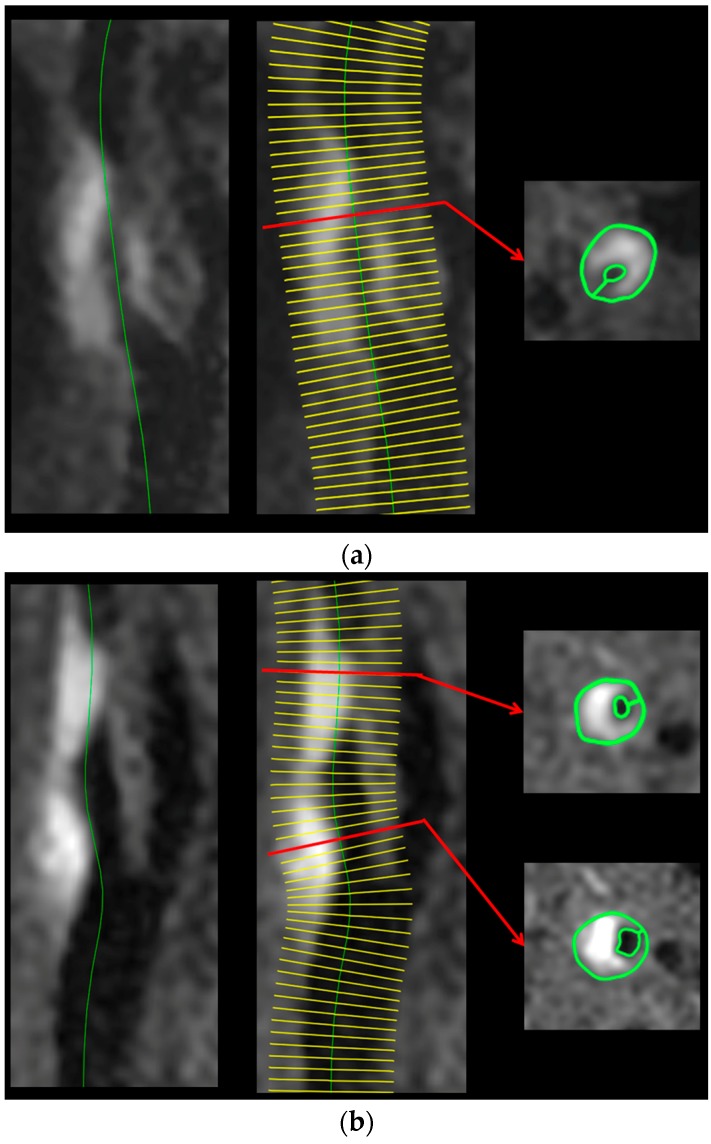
Preoperative three-dimensional fast spin echo T1-weighted magnetic resonance (3D FSE T1-weighted MR) plaque images of two patients with MES during exposure of the carotid arteries in endarterectomy. (a) The curved planar reformation is performed parallel to the long axis of the common and internal carotid arteries (left). The green line indicates the center line of the vessel lumen. On the image of the curved planar reformation, axial images with 1.0 mm thickness are reformatted as sections perpendicular to the center line (middle). Yellow lines indicate the position of each reformatted axial image. A reformatted axial image with the maximum POR and maximum plaque intensity (red arrows) are determined by tracing a plaque and vessel lumen of the common or internal carotid artery (right, green lines). A 65-year-old man with asymptomatic right internal carotid artery stenosis exhibits identification of the image with the maximum POR and the image with the maximum plaque intensity; (b) Preoperative 3D FSE T1-weighted MR plaque images of two patients with MES during exposure of the carotid arteries in endarterectomy. The curved planar reformation is performed parallel to the long axis of the common and internal carotid arteries (left). The green line indicates the center line of the vessel lumen. On the image of the curved planar reformation, axial images with 1.0 mm thickness are reformatted as sections perpendicular to the center line (middle). Yellow lines indicate the position of each reformatted axial image. A reformatted axial image with the maximum POR and maximum plaque intensity (red arrows) are determined by tracing a plaque and vessel lumen of the common or internal carotid artery (right, green lines). A 77-year-old man with symptomatic right internal carotid artery stenosis exhibits no identification of the image with the maximum POR (upper) and the image with the maximum plaque intensity (lower).
